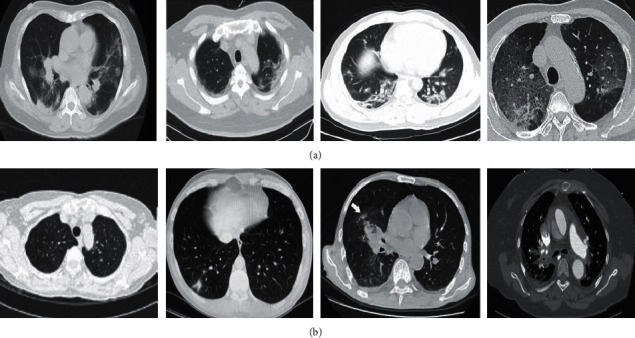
(a) Examples of positive COVID19 CT scan images. (b) Examples of non-COVID19 CT scan images.
